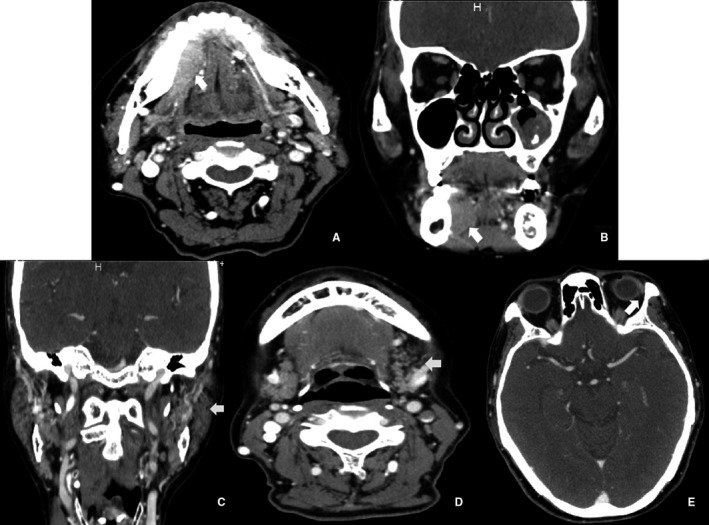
Enhanced computed tomography suggested MALT lymphoma in the right sublingual gland (70‐year‐old female). A spindle‐shaped soft‐tissue mass with an unclear boundary was observed in the right sublingual gland (A, B). Bilateral parotid glands and submandibular glands were uneven with spotted high‐density focus, considered as a lymphoepithelial lesion or SS. The left sublingual gland and bilateral lacrimal glands were atrophic (C–E)
